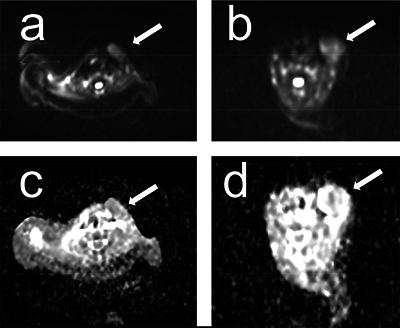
Diffusion weighted imaging (DWI b800) performed in patients 3 and 4 (parts a and b, respectively) showing hyperintensity of the fibromatosis colli (arrows) with accompanying low ADC values ranging from 1.25 to 1.4.
